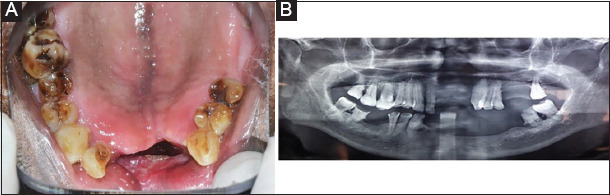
(A) Intraoral view of premaxillary defect. (B) Orthopantomagram.
